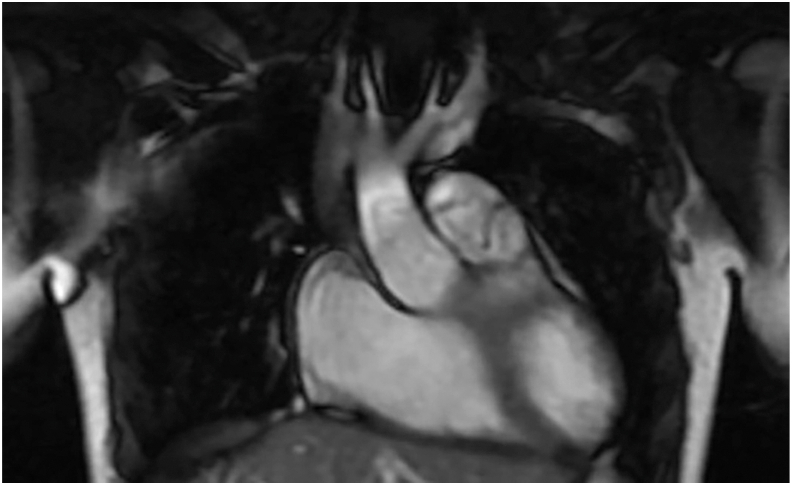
Preoperative cardiac magnetic resonance image, coronal view, showing discrete pulmonary valve leaflets.
